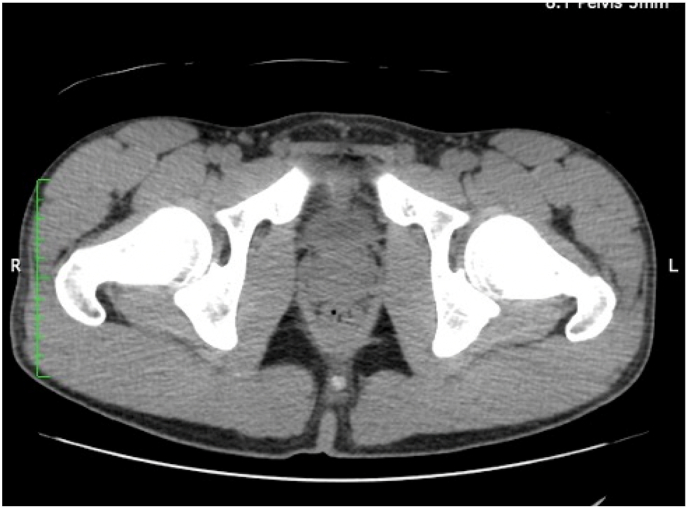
Abdominal enhanced computed tomography. Enhanced computed tomography (CT) of the abdomen showed no sign of inguinal hernia.
